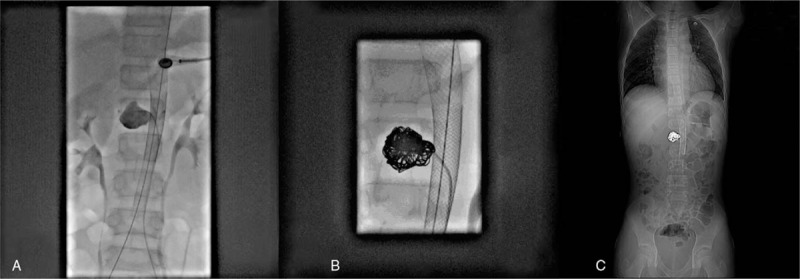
A stent implanted in the aorta and radiography showed the aneurysm (A). Eight spring coils were imbedded (B). The CT scout image showed the stent and spring coils after aneurysm embolization (C).
